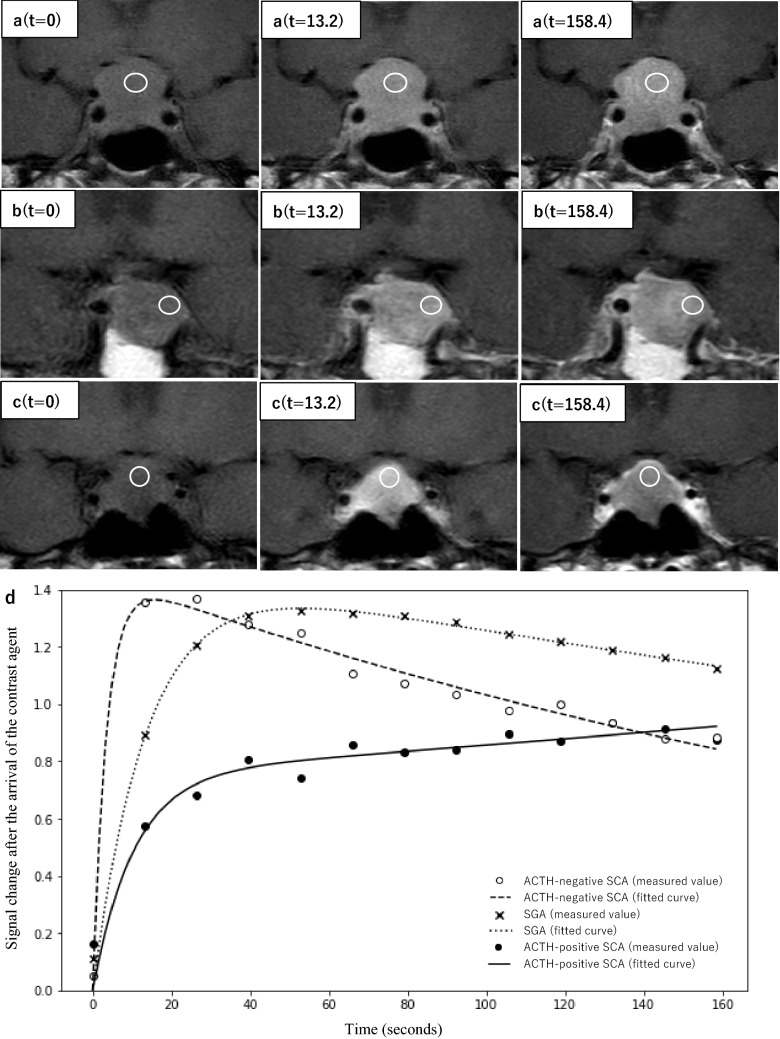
The initial dynamic MRI phase and late phase images. a A 36-year-old man with adrenocorticotropic hormone (ACTH)-positive silent corticotroph pituitary adenoma (SCA). b A 77-year-old woman with silent gonadotroph adenoma (SGA). c A 46-year-old woman with ACTH-negative SCA. Dynamic MR signal intensity values are measured in a circular or oval region of interest (ROI) where the highest signal increase is observed within each lesion. The ROI is copied to all the phases (white circle). The ACTH-positive SCA case shows a relatively more-gradual contrast in the early phase than the SGA case (a, b). In contrast, the ACTH-negative SCA case shows relatively more-rapid contrast in the early phase than the SGA case (b, c). The time-intensity curve of this case is shown (d). For the ACTH-positive SCA case, the measured values are plotted as black circles, and the fitted curve is shown as a solid line; the time-intensity curve has a more gradual rise than the SGA case (measured values are plotted as a cross; the fitted curve is shown as a dotted line). For the ACTH-negative SCA case, the measured values are plotted as white circles, and the fitted curve is shown as a dashed line; the time-intensity curve has a more rapid rise than the SGA case
